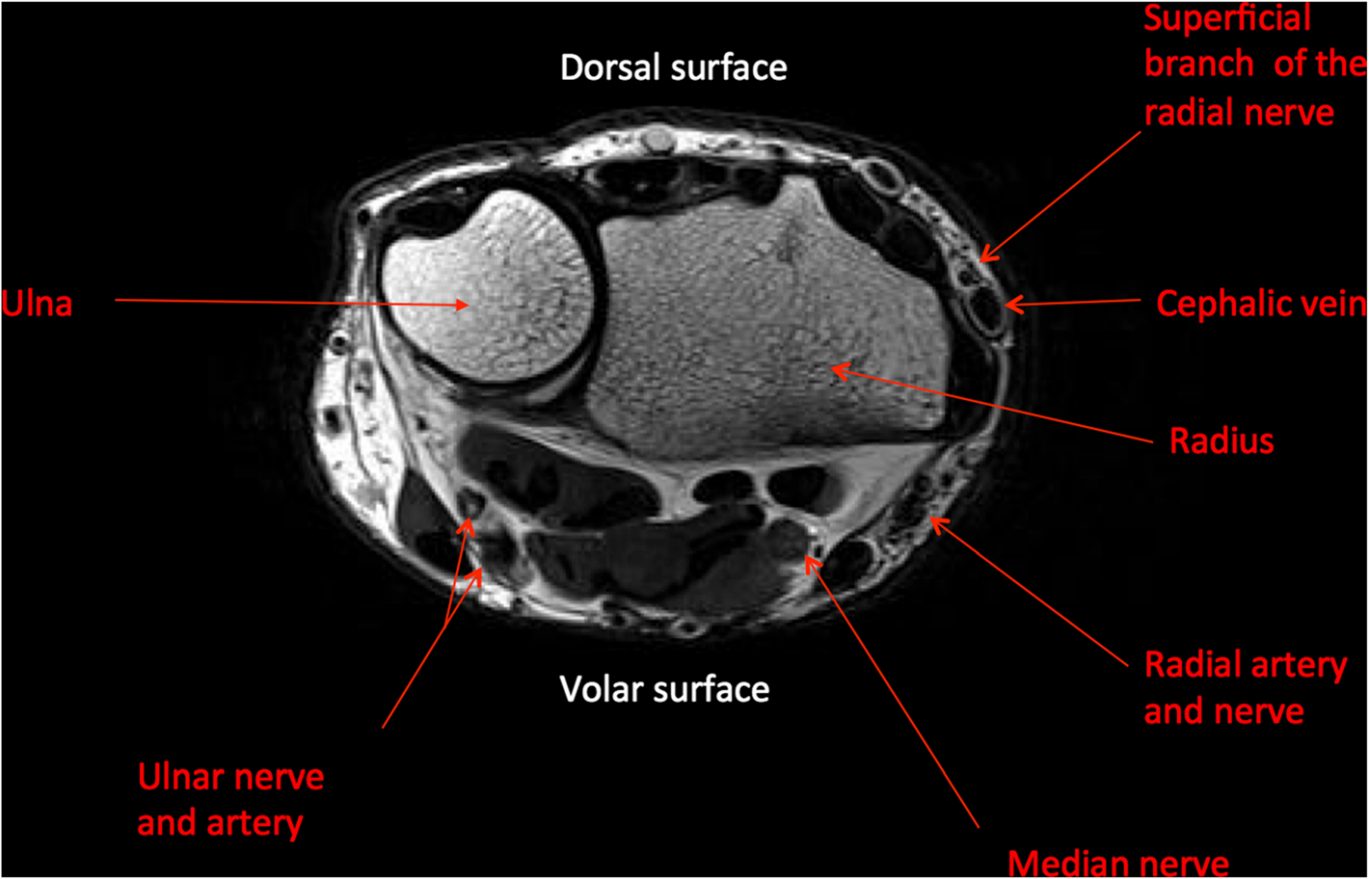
Wrist magnetic resonance imaging anatomy. T1-weighted axial view demonstrating the specific proximity of the ulnar, radial and median nerves to the skin
